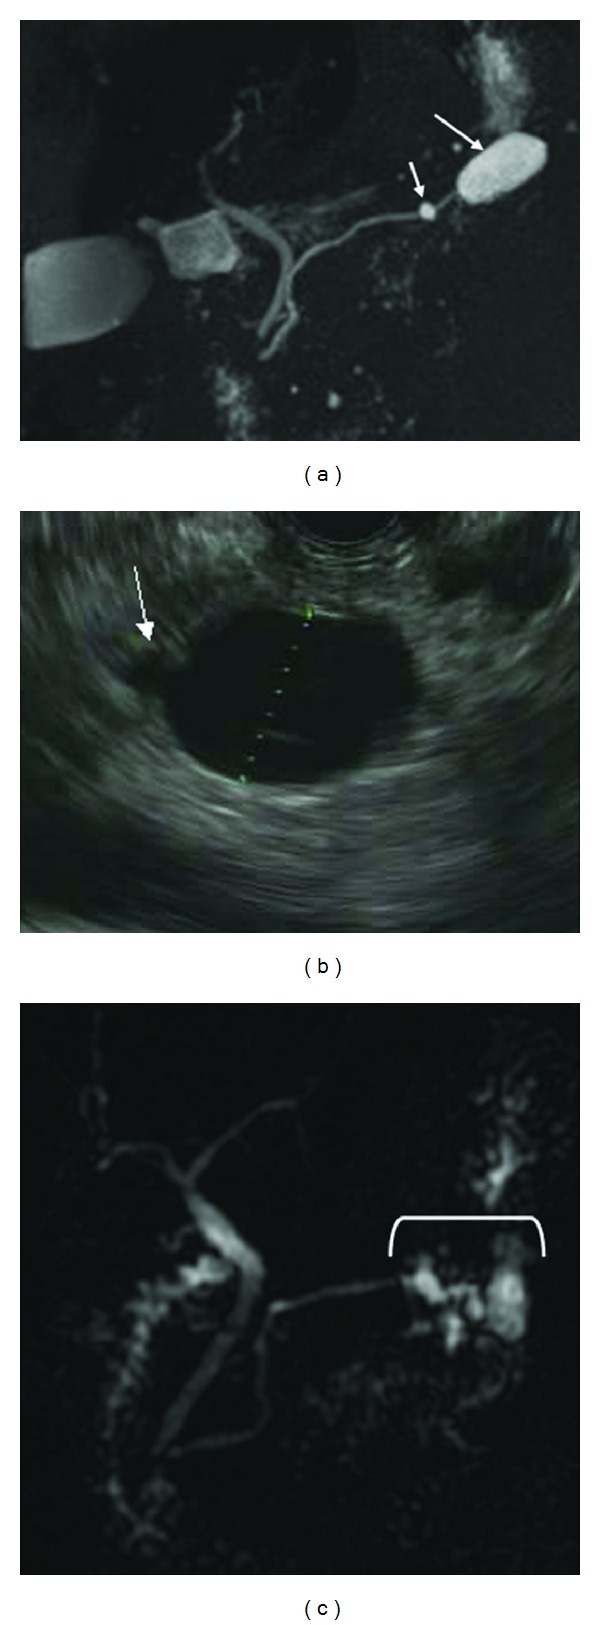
Imaging of BD-IPMN and mixed IPMN. (a) MRI of BD-IPMN: arrow points to communication between BD-IPMN and normal main pancreatic duct. (b) EUS of BD-IPMN: arrow points to communication between cyst and main pancreatic duct. (c) MRI of mixed IPMN: arrow points to diffusely massively dilated main pancreatic duct.
